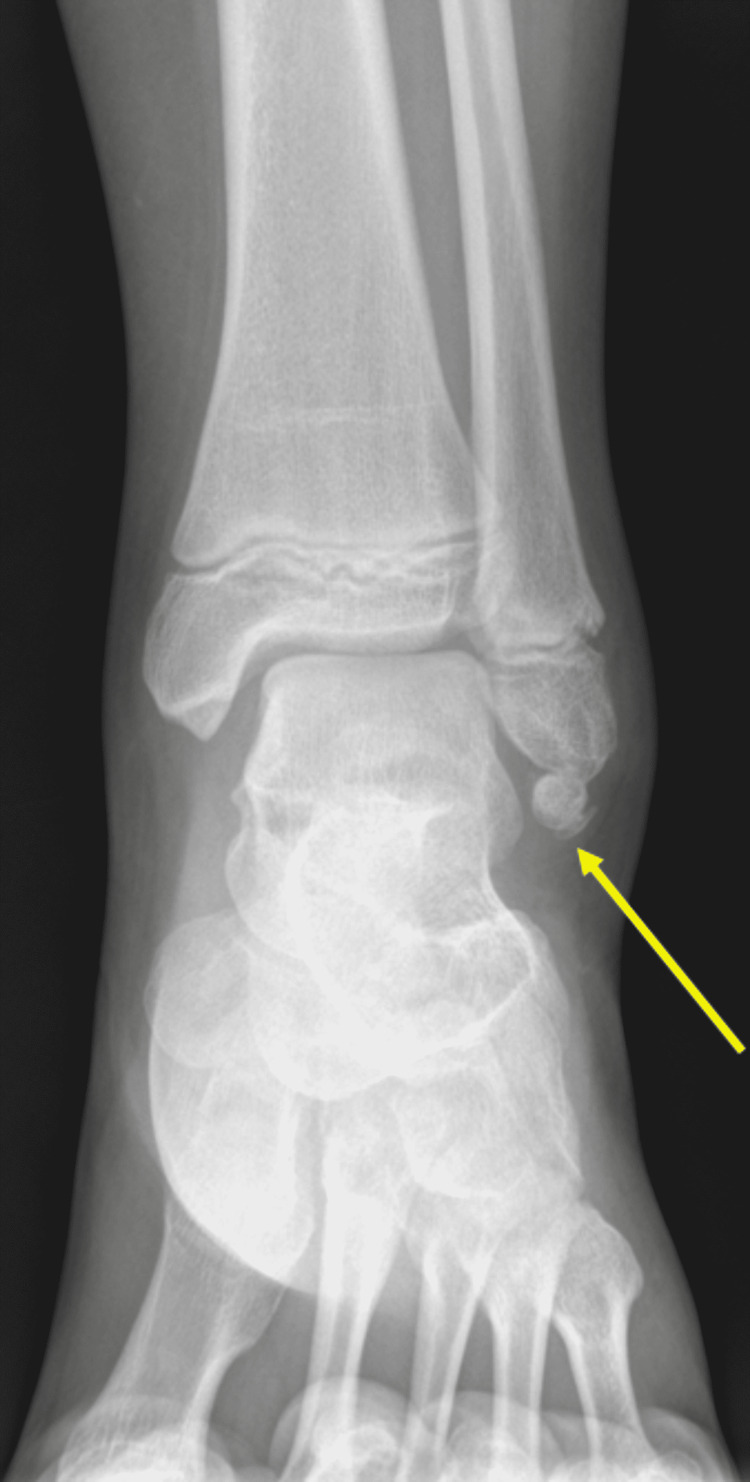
AP radiograph of the left ankle shows ossified fragments at the distal lateral malleolus (yellow arrow), similar to the appearance on prior radiographs. AP: anterior-posterior.
